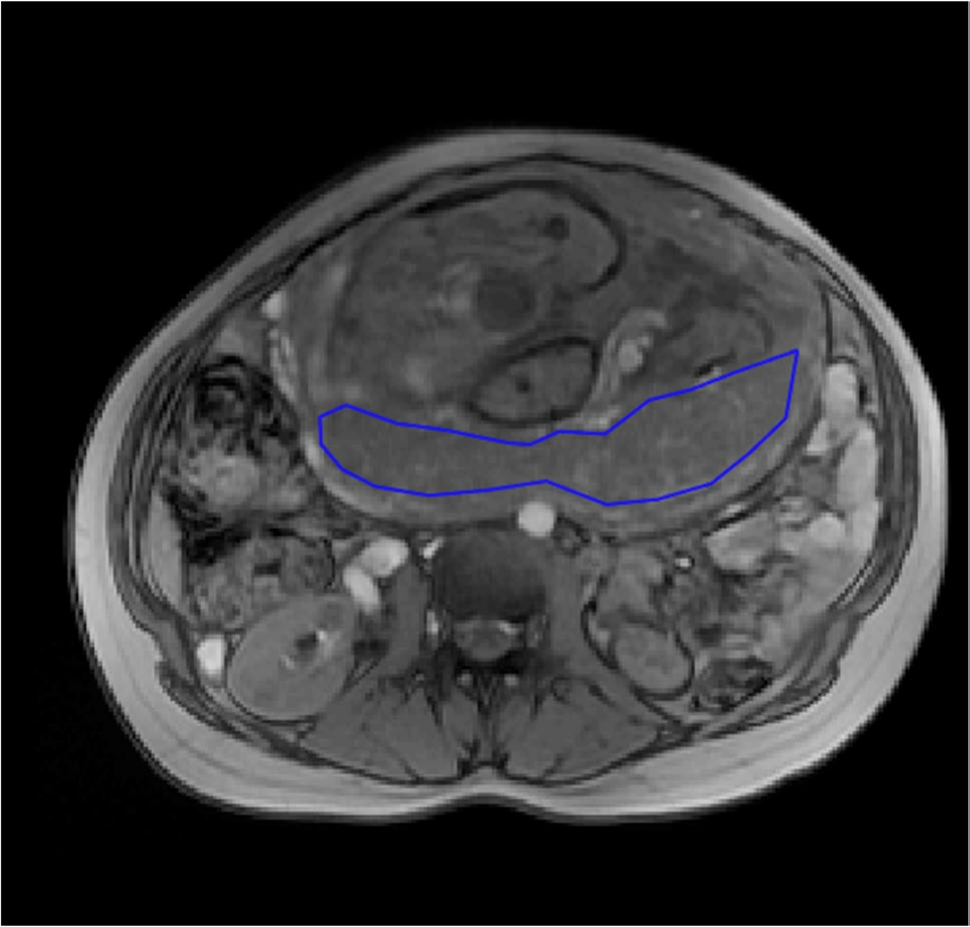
T2*-weighted magnetic resonance image of a transverse section of a pregnant woman, with the outline of the placenta (the region of interest) drawn up
